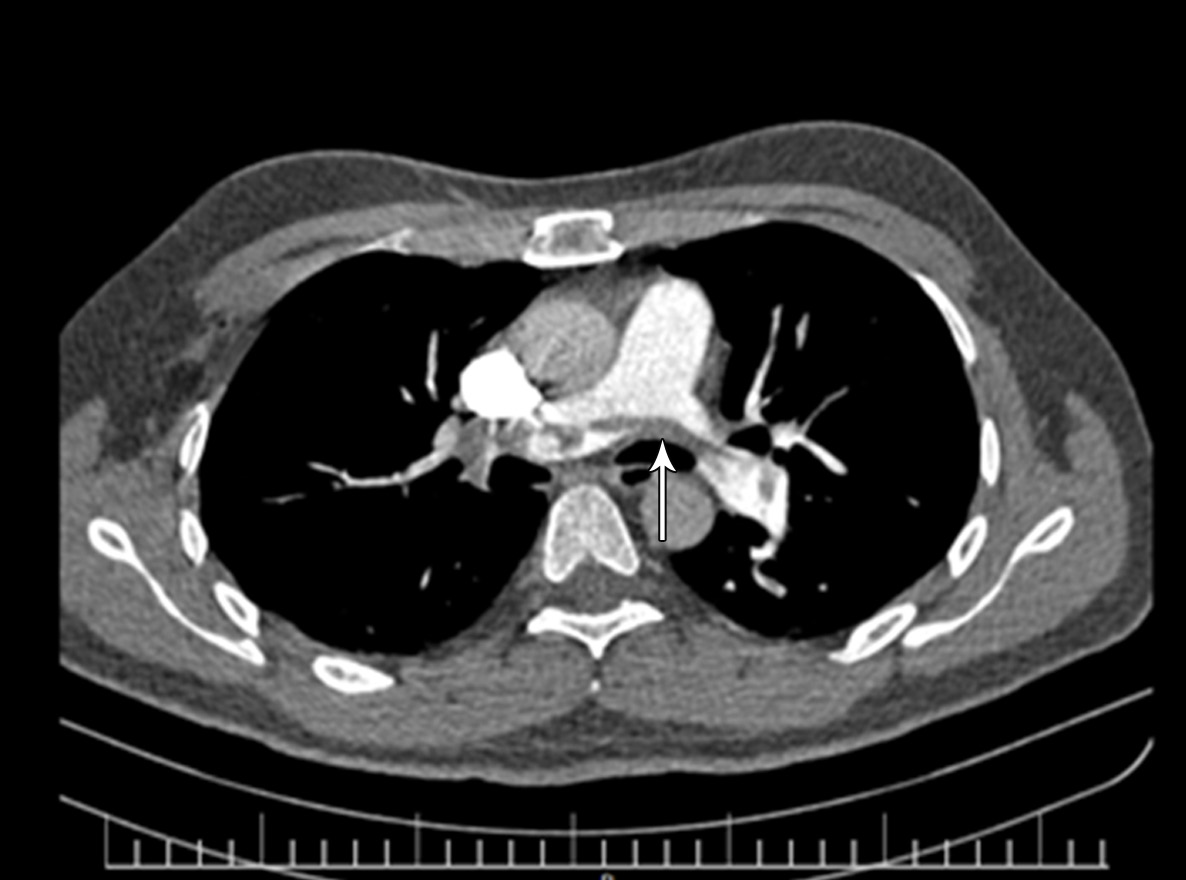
Bilateral massive pulmonary thromboembolism (arrow) in multi-detector computed tomography angiography
